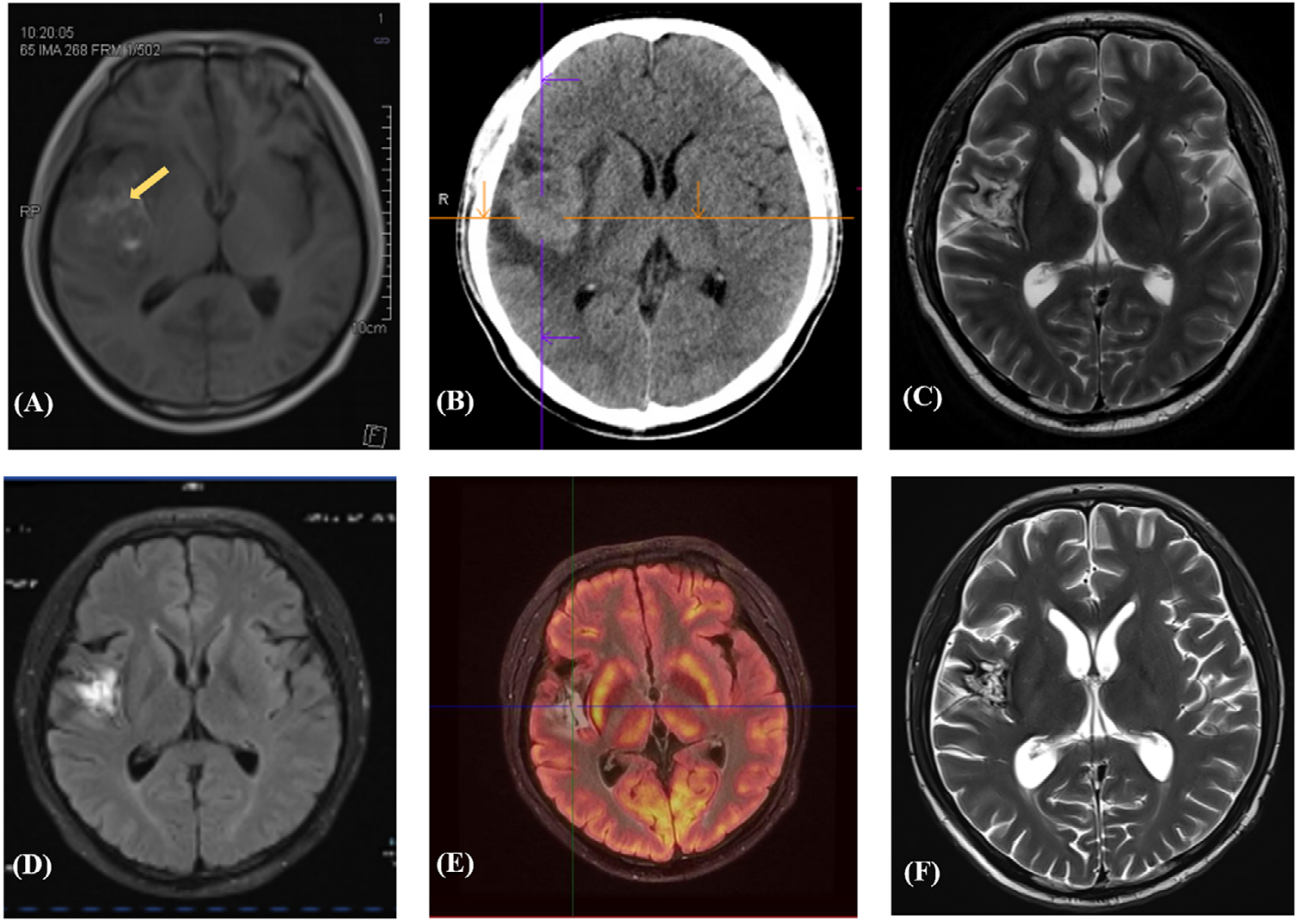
Brain images of central nervous system‐post‐transplant lymphoproliferative disorder (CNS‐PTLD). (A) Emergence of CNS‐PTLD. Positron emission tomography‐computed tomography (PET‐CT) revealed unevenly increased lesions in fluorodeoxyglucose (FDG) metabolism in the right temporal lobe (yellow arrow). The lesion measured was approximately 4.3 × 3.9 × 4.0 cm, SUVmax of 8.0, and central metabolism with SUVmax of 4.7. (B) Following R‐MD+orelabrutinib treatment for CNS‐PTLD. FDG metabolism in the right temporal lobe displayed irregularly heightened levels and a mixed‐density mass, consistent with lymphoma combined with hemorrhage. The intracranial lesions were smaller in size and slightly higher in metabolic activity than before: 1. the lesion measured was approximately 3.6 × 3.1 × 3.7 cm; 2. FDG uptake was unevenly increased with nodular‐like elevation, with SUVmax of 10.8 at the edge and slightly decreased central metabolism with SUVmax of 4.3. (C) Following immunochemotherapy +WBRT, nodular and patchy mixed signal shadows were observed in the right parietal lobe, indicating no further hemorrhage. (D) Three months after WBRT, abnormal signal shadows were visible in the right temporal lobe, with irregular morphology, measuring approximately 1.9×1.7×3.4 cm, which demonstrated partial remission. (E) After one cycle of TEDDi‐R treatment, abnormal signal shadows were observed in the right insular and temporal lobes, with no significant increase in FDG uptake, achieving CMR in the patient. (F) No significant enhancement was observed in the lesion by contrast‐enhanced MRI, which was similar to the MRIs on May 14, March 5, February 16, 2024, and November 10, 2023 (See Figure S1).
